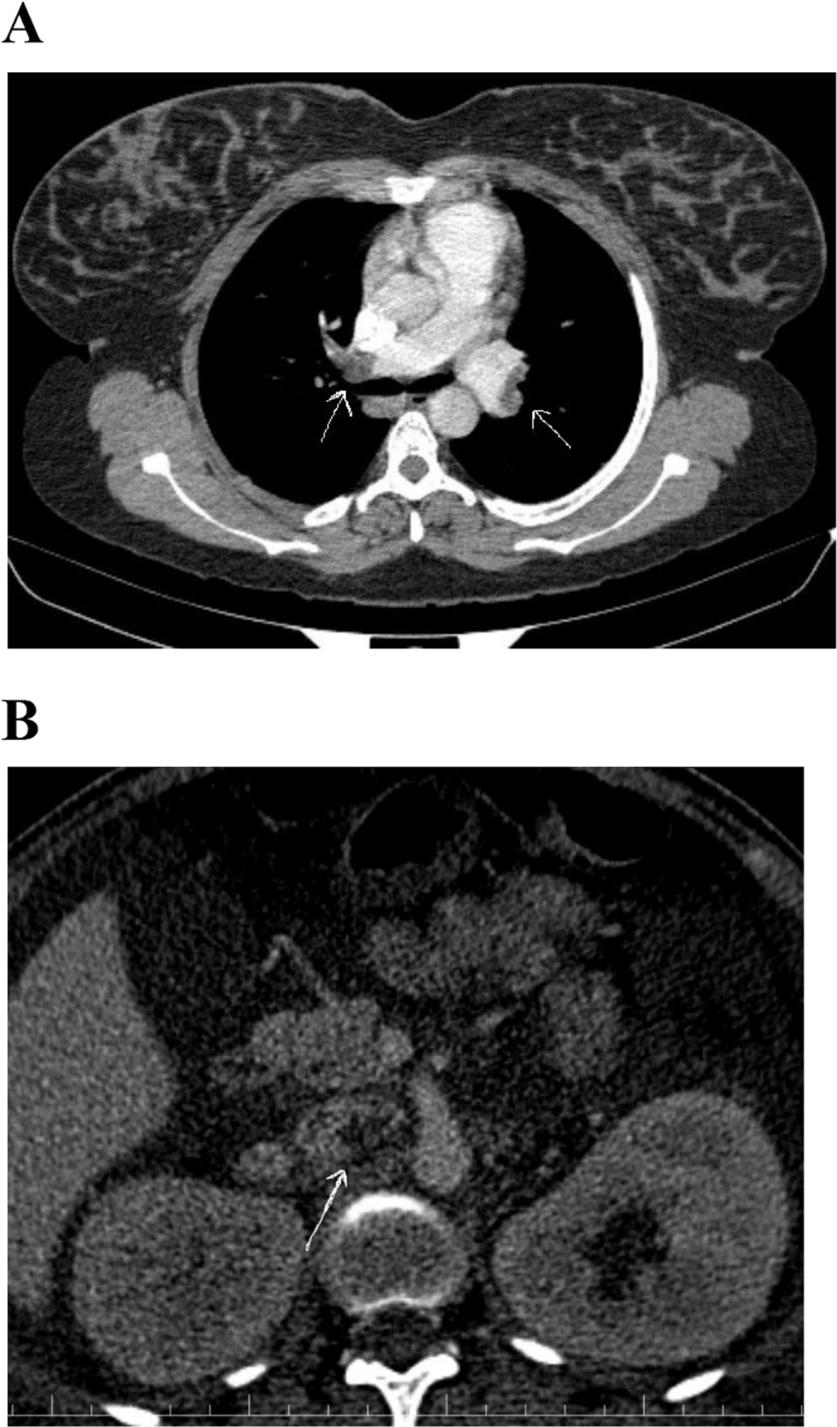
A Computed tomography pulmonary angiography revealed intraluminal filling defects representing emboli in the right and also left pulmonary arteries (PE). B An abdominal CT scan showed thrombus in the inferior vena cava
